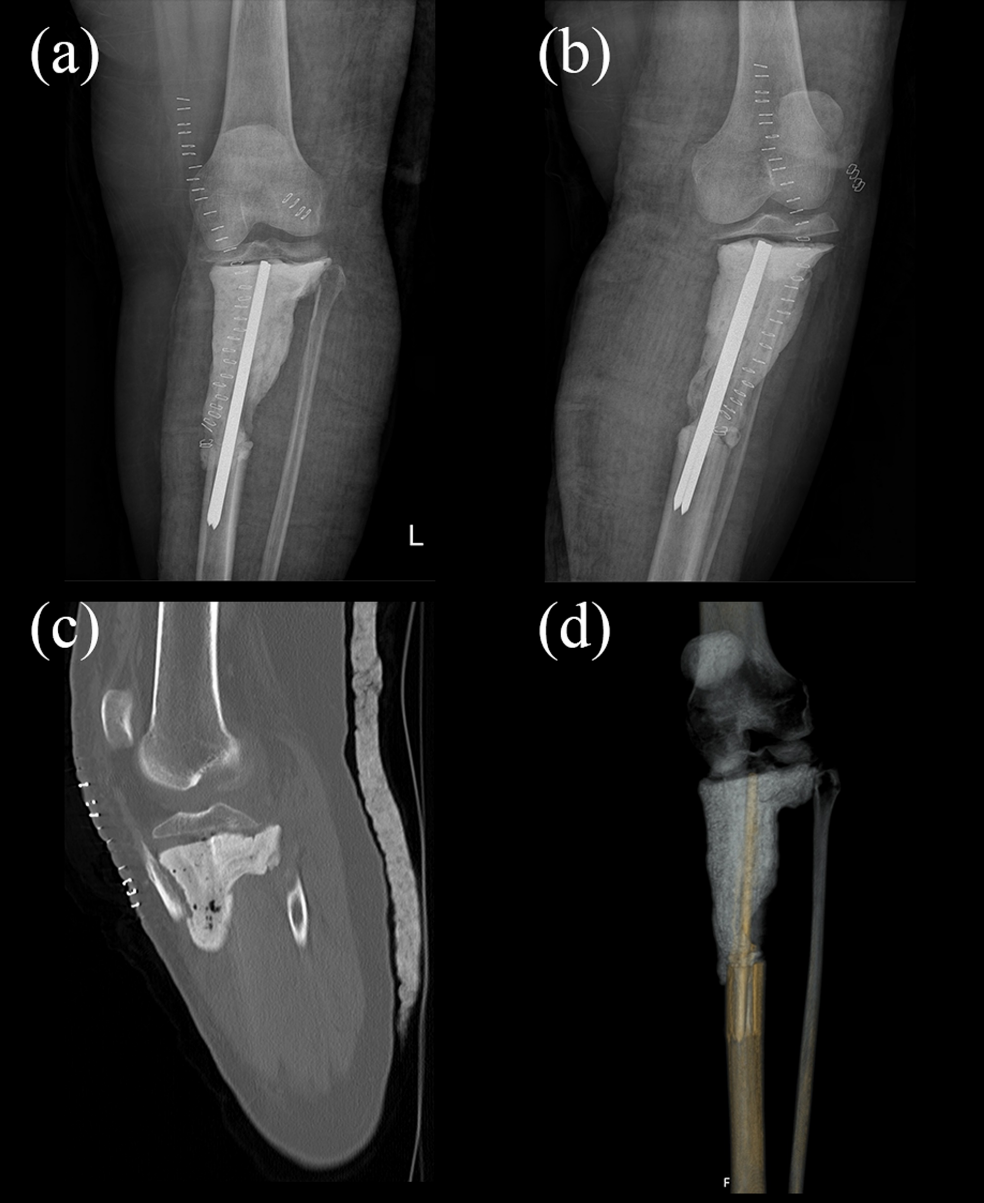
Postoperative imaging of the patient (a-b) Postoperative X-rays; (c,d) postoperative CT images CT: computed tomography
